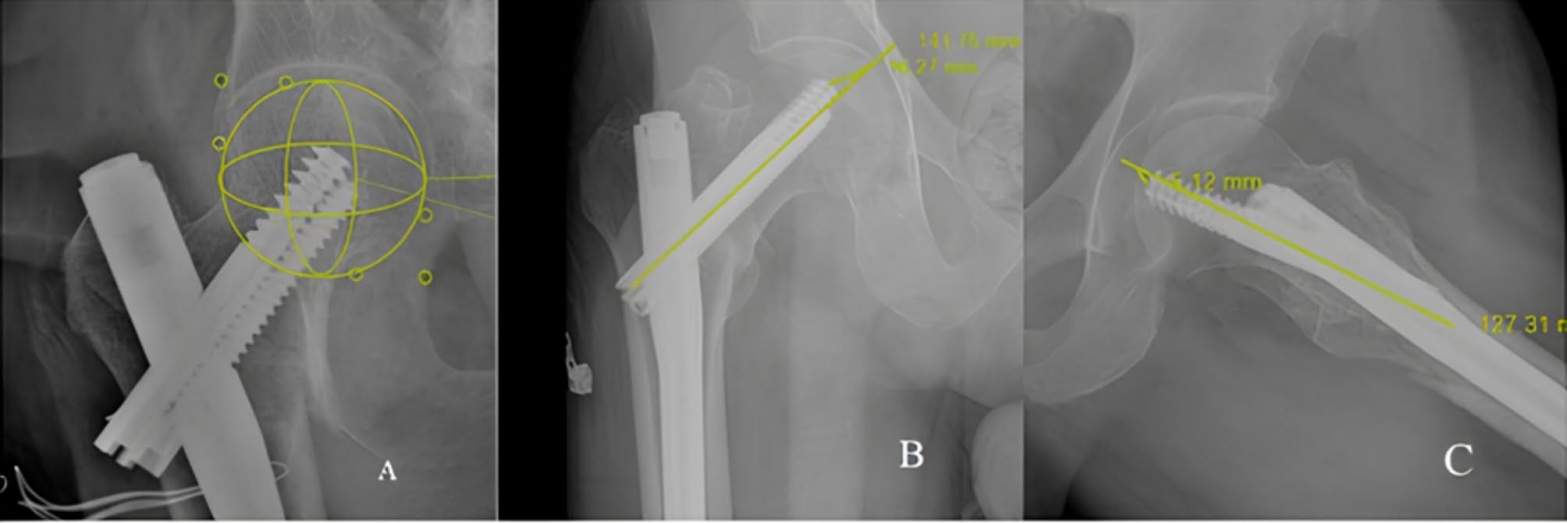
Examples of radiographic evaluation of PFN fixation. (A) Cleveland zone. (B) AP X-ray; TAD. (C) Lateral X-ray; TAD
